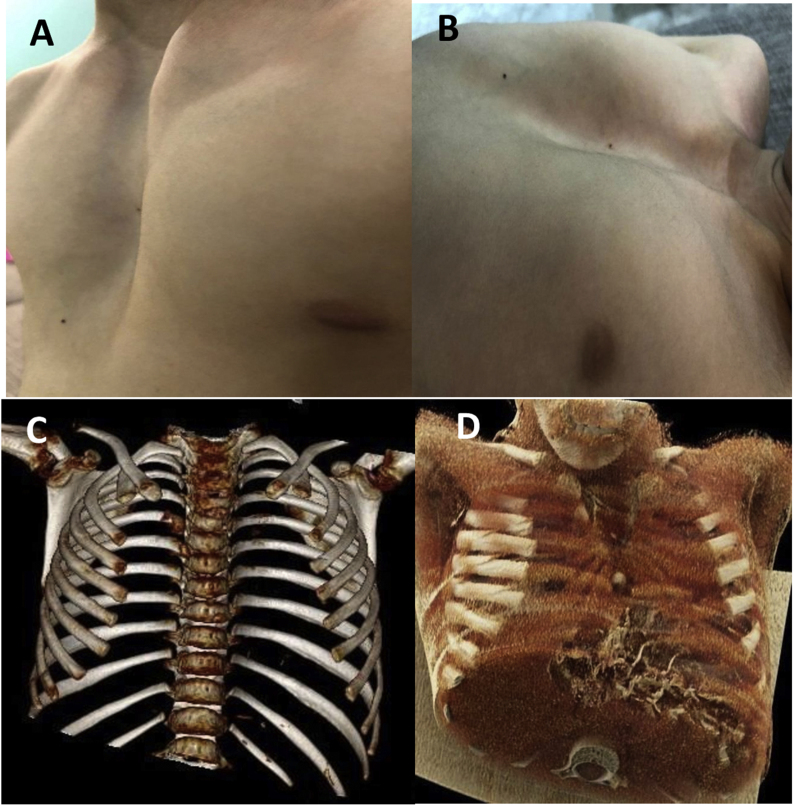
A. Patient's photograph in standing position showing wide sternal gap. B. Patient's photograph in supine position showing wide sternal gap. C & D. 3D CT scan of Thorax showing congenitally absent sternum.
